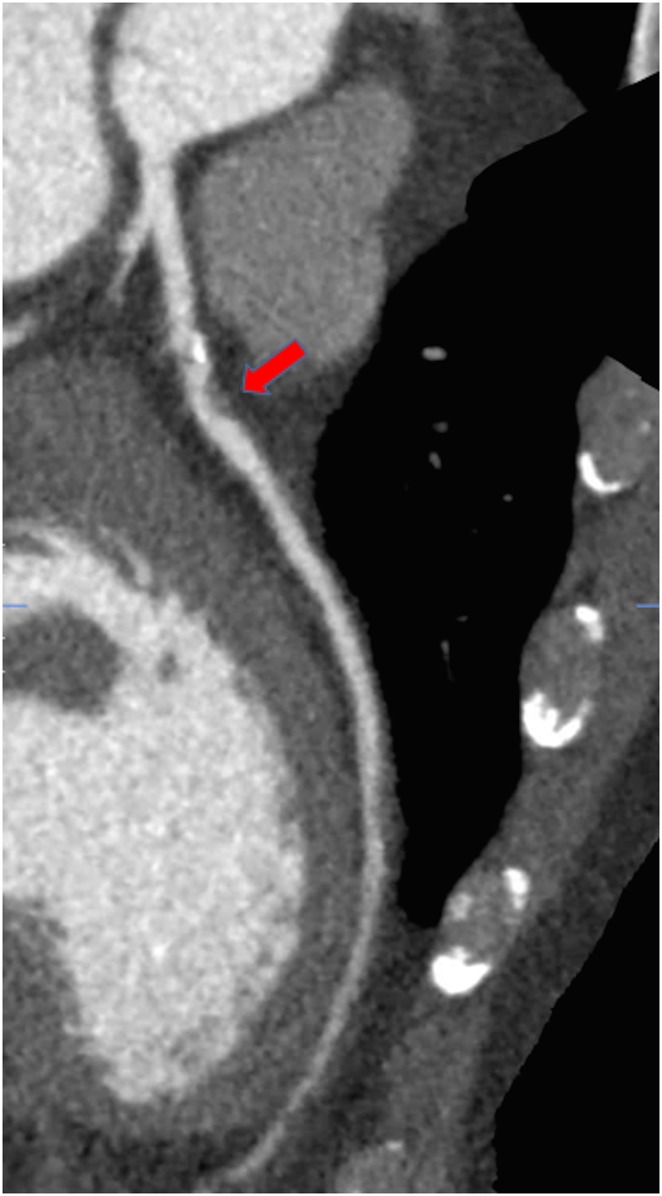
Computed tomography coronary angiography image of a plaque with high-risk characteristics including low attenuation (red arrow).
